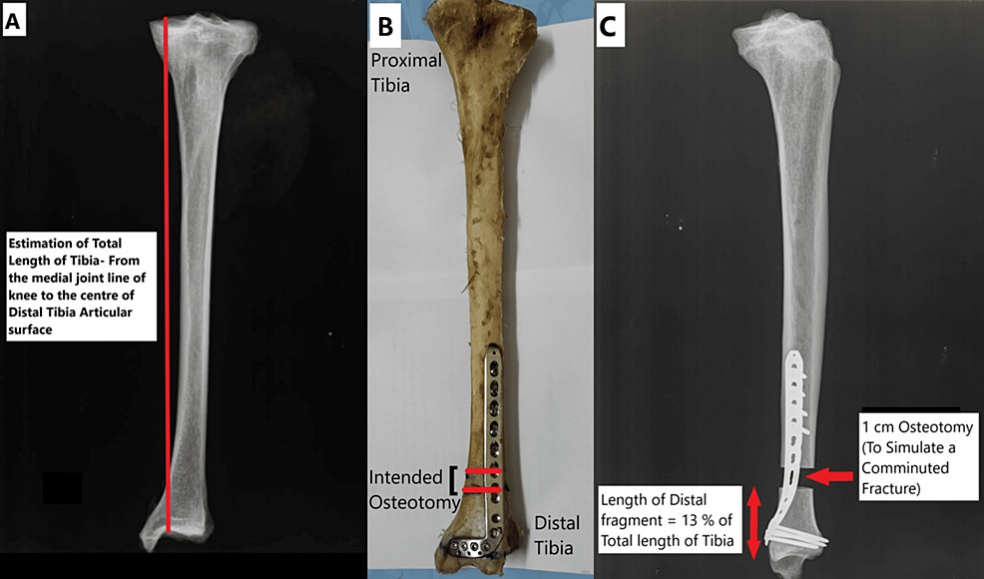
(A) Method of estimation of the total length of tibia. (B) Depiction of the posterior aspect of the tibia with the marked site of intended osteotomy. (C) Post-osteotomy radiograph demonstrating the length of the distal fragment.
